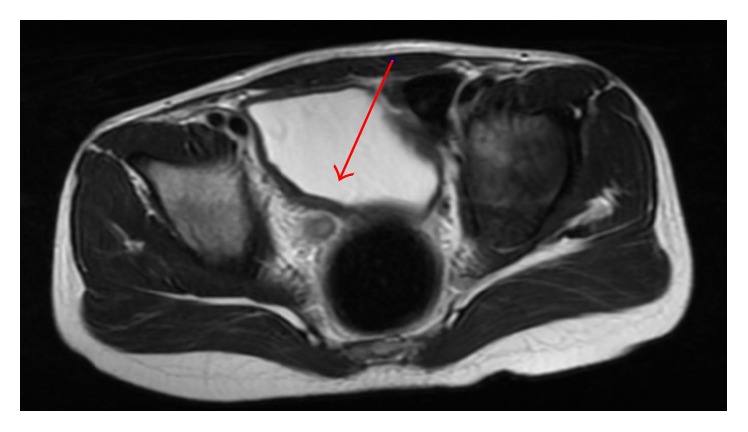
Axial T2-weighted MRI after one month showed discrete bladder wall thickening with homogenous low signal intensity (arrow).
